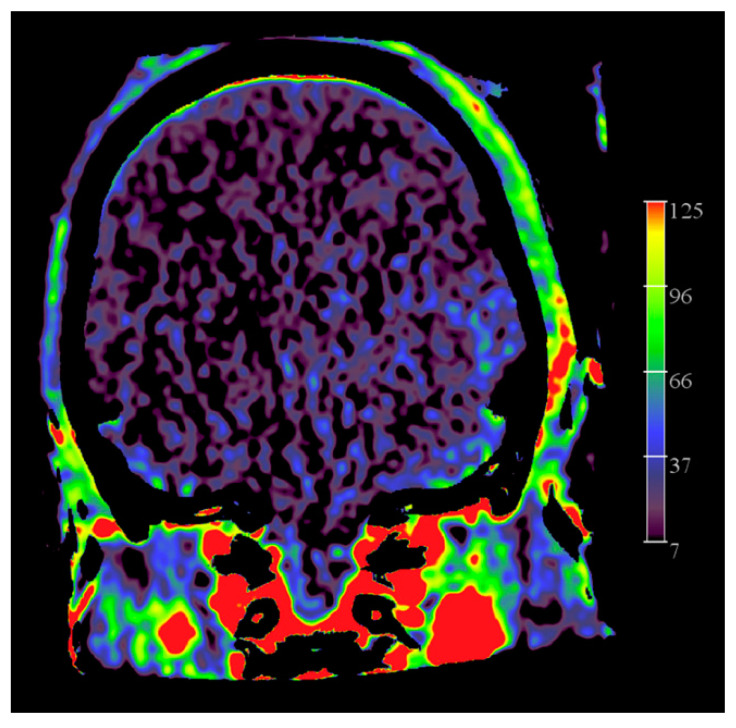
FD CT PBV imaging in a patient with no intracranial flow. Coronal PBV MIP reformation demonstrates filling of the ECA vascular territories, including middle meningeal artery, but no filling of pial arteries.
